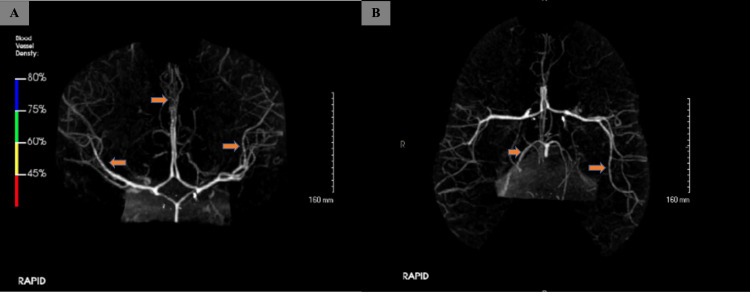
CTA of the brain A: Mild to moderate diffuse narrowing of the ACAs and MCAs (orange arrows); B: Mild to moderate diffuse narrowing of MCAs and PCAs (orange arrows) CTA: Computed tomography angiography, ACAs: Anterior cerebral arteries, MCAs: Middle cerebral arteries, PCAs: Posterior cerebral arteries
